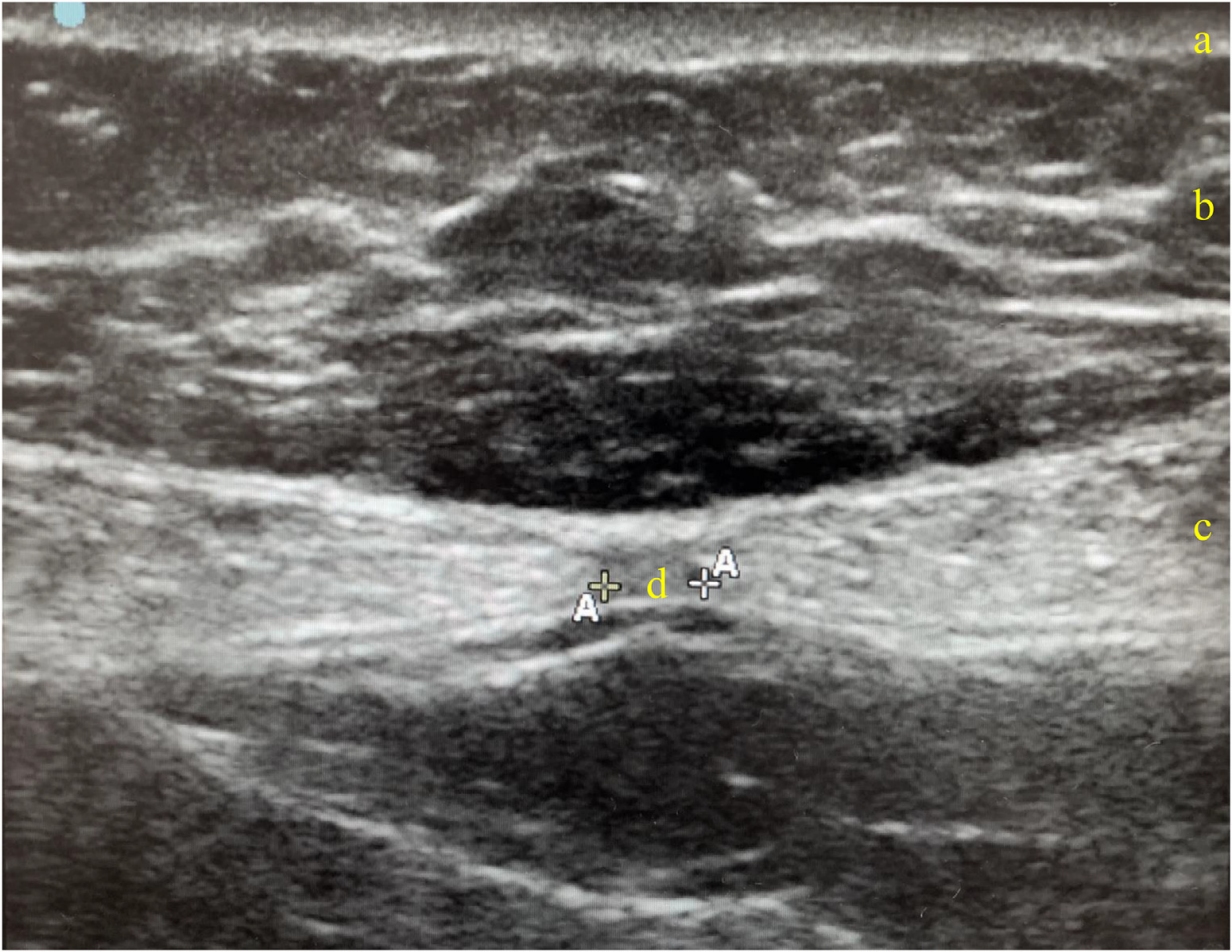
Ultrasound measurement of the IRD. (a) Dermis layer; (b) Subcutaneous tissue layer; (c) Rectus abdominis muscles layer; (d) IRD measurement, defined as the distance between the medial edges of the rectus abdominis muscles: A landmarks visible on the screen.
